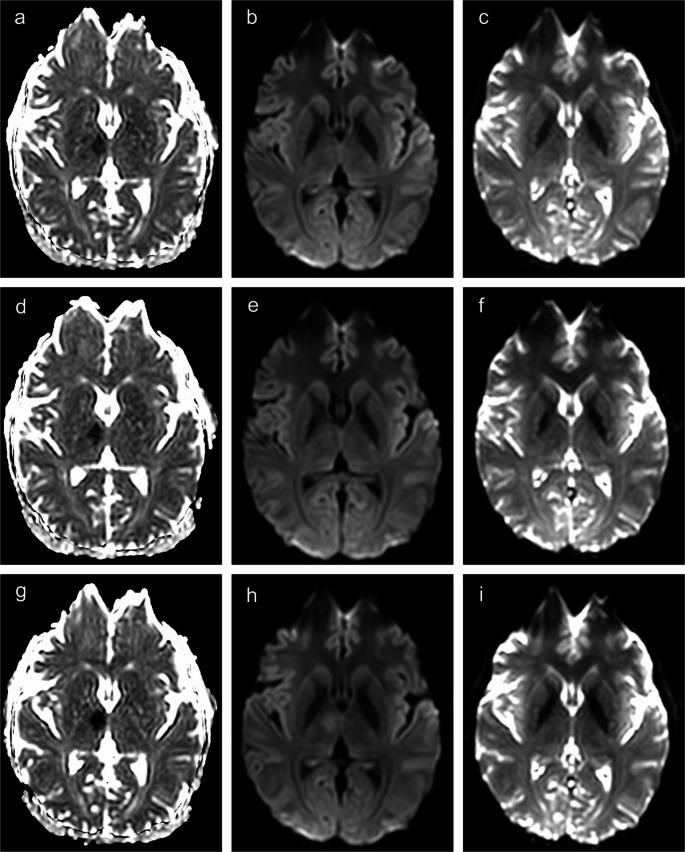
Sequential ADC (left) and DWI (middle) signal changes in a right ventromedial thalamic ischemic lesion, with corresponding b = 0 images (right). Baseline (a–c), after 6 min (d–f), and after 3.5 h (g–i)
